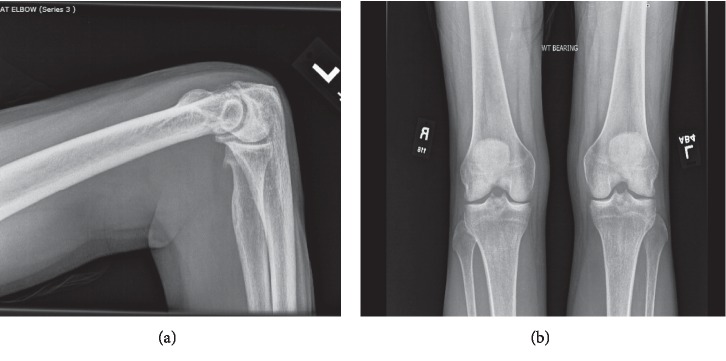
X-ray of the left elbow joint and bilateral knee joints. (a) Moderate osteophytosis at the humeroulnar articulation and involving the radial head with mild joint space narrowing and no joint effusion. (b) Anterior-posterior weight-bearing view; no acute fracture/dislocation, and joint spaces grossly preserved.
